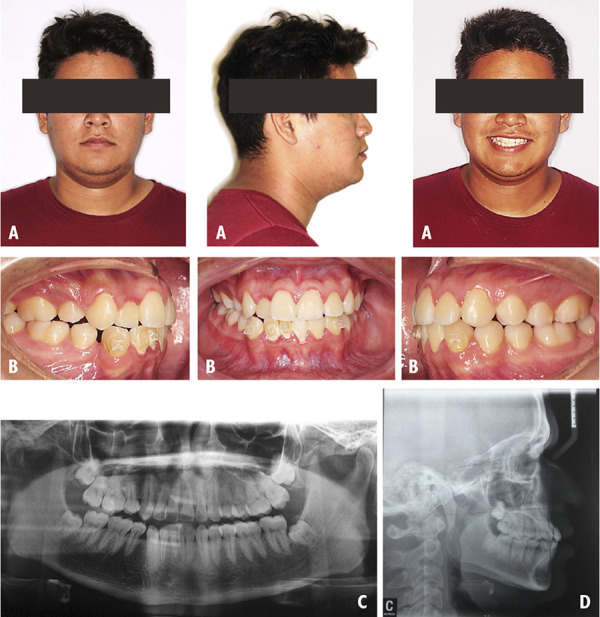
Case 3. Initial pre-treatment records. A: Extraoral photographs. B: Intraoral photographs. C: Panoramic radiograph. D: Cephalometric radiograph.
